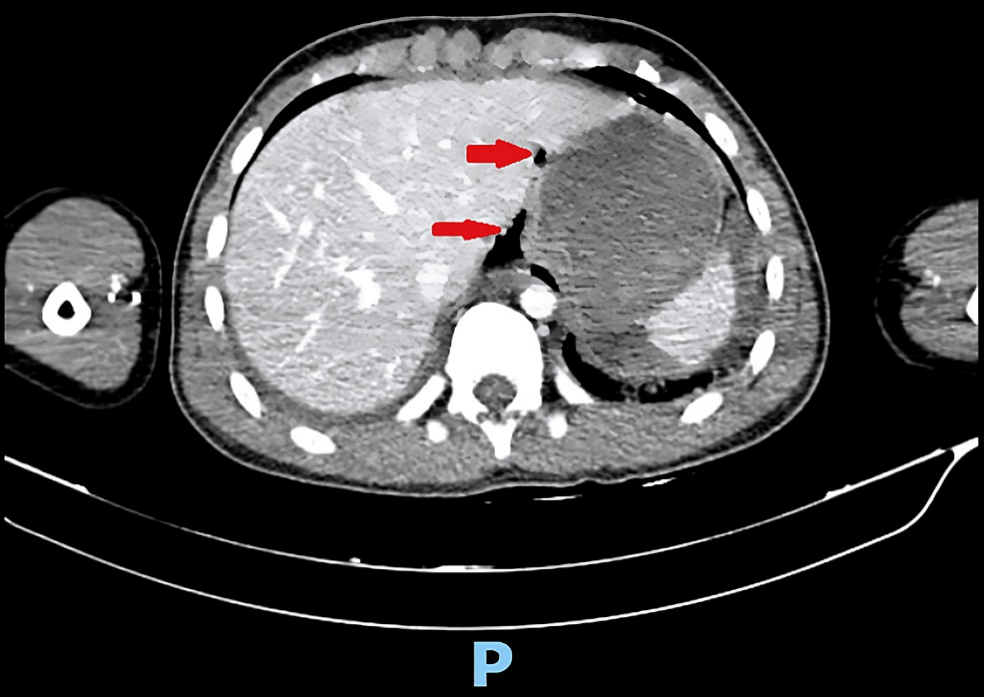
Axial view from the initial CT with IV contrast of the chest showing the esophagus surrounded by fluid and air (signs of esophageal disruption).
